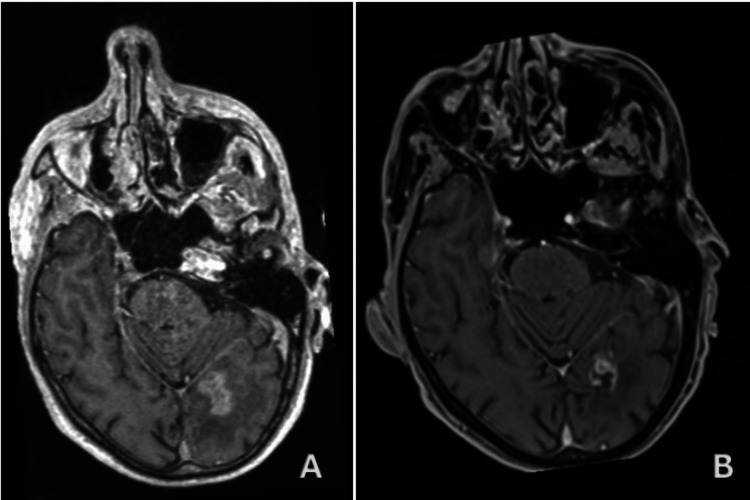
Patient A, pre- and post-GRS imaging (A) Initial imaging of recurrent metastatic lesion on pre-treatment axial T1 post-contrast MRI. (B) Complete response on post-treatment axial T1 post-contrast MRI at 17 months follow-up. GRS: gyroscopic stereotactic radiosurgery
